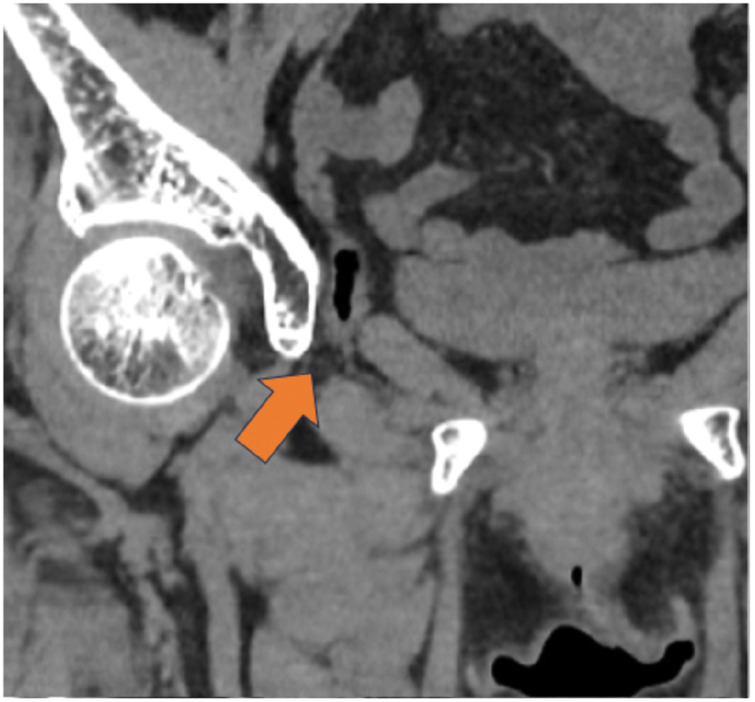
The initial CT scan performed at the referring hospital. Arrow: herniation of the small intestine through the obturator foramen.
